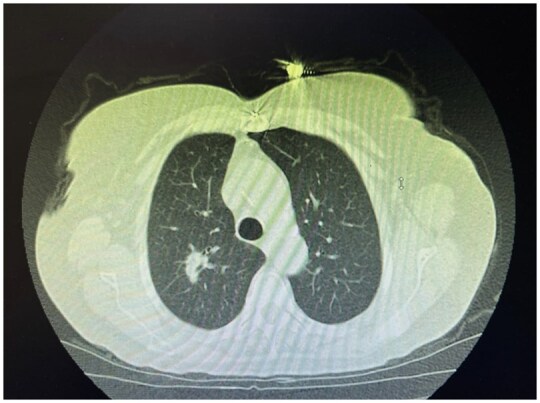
Axial chest CT image showing a nodular lesion in the posterior segment of the right upper lobe.
